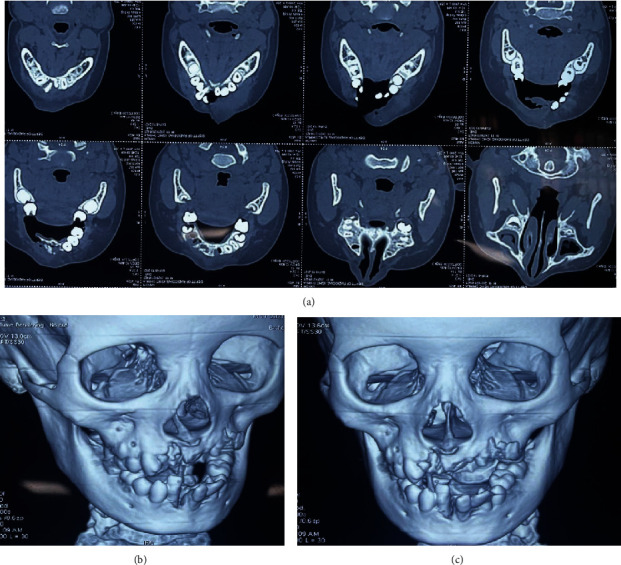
(a–c) Computed tomography (CT) scan showing multiple avulsions along with alveolar bone fracture.
